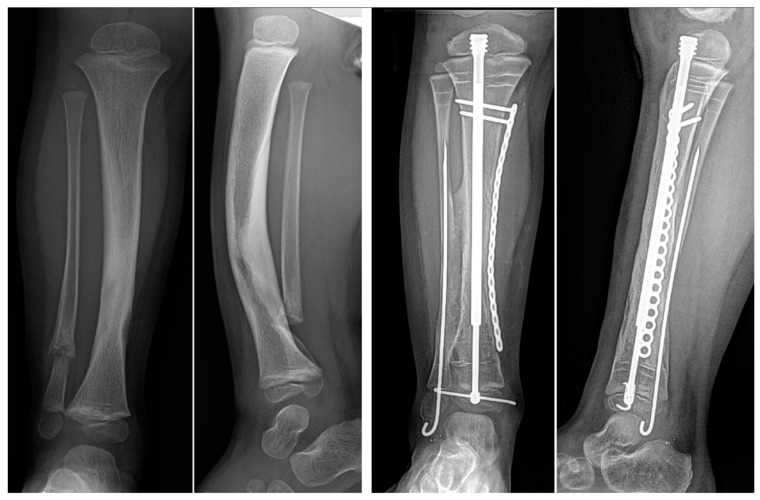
AP and lateral radiographs of a patient with Paley type 2A CPT. The preoperative images on the left show an intact tibia with fibular pseudarthrosis. A tibial osteotomy was made at the apex of the deformity, straightened, and fixed with the standard cross-union protocol. The postoperative images on the right show a well-healed osteotomy and cross-union with normal tibial alignment.
